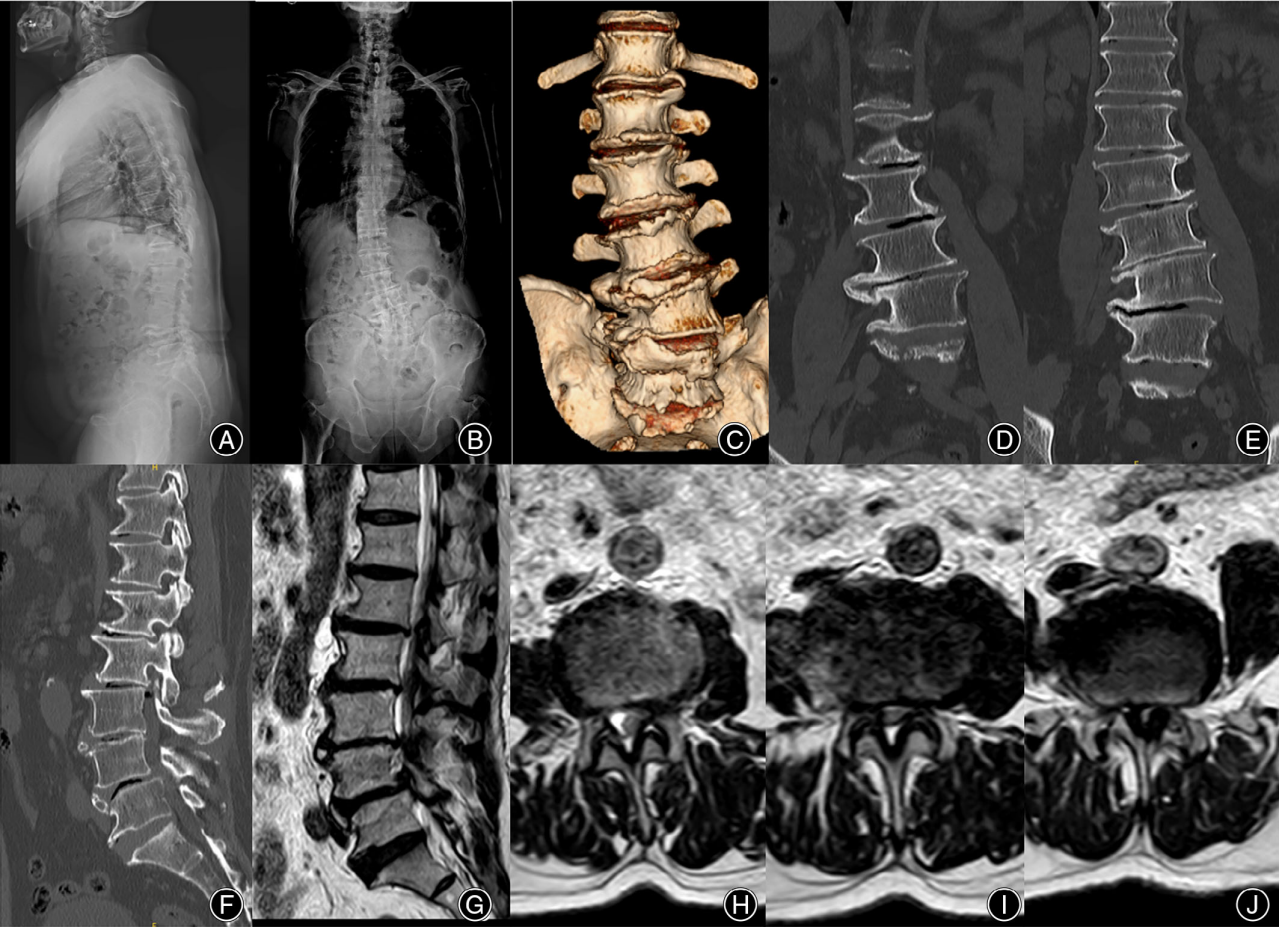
Whole spine X‐rays showed lumbar scoliosis with coronal and sagittal imbalance (A, B). CT showed lumbar degenerative spondylolisthesis (L4), with an osteophyte bridge at the right lateral intervertebral space of L_3/4_ and L_4/5_ (C–F). MRI showed severe spinal stenosis at L_3/4_ and L_4/5_ segments, and Schizas grade was C and D, respectively (G–J).
